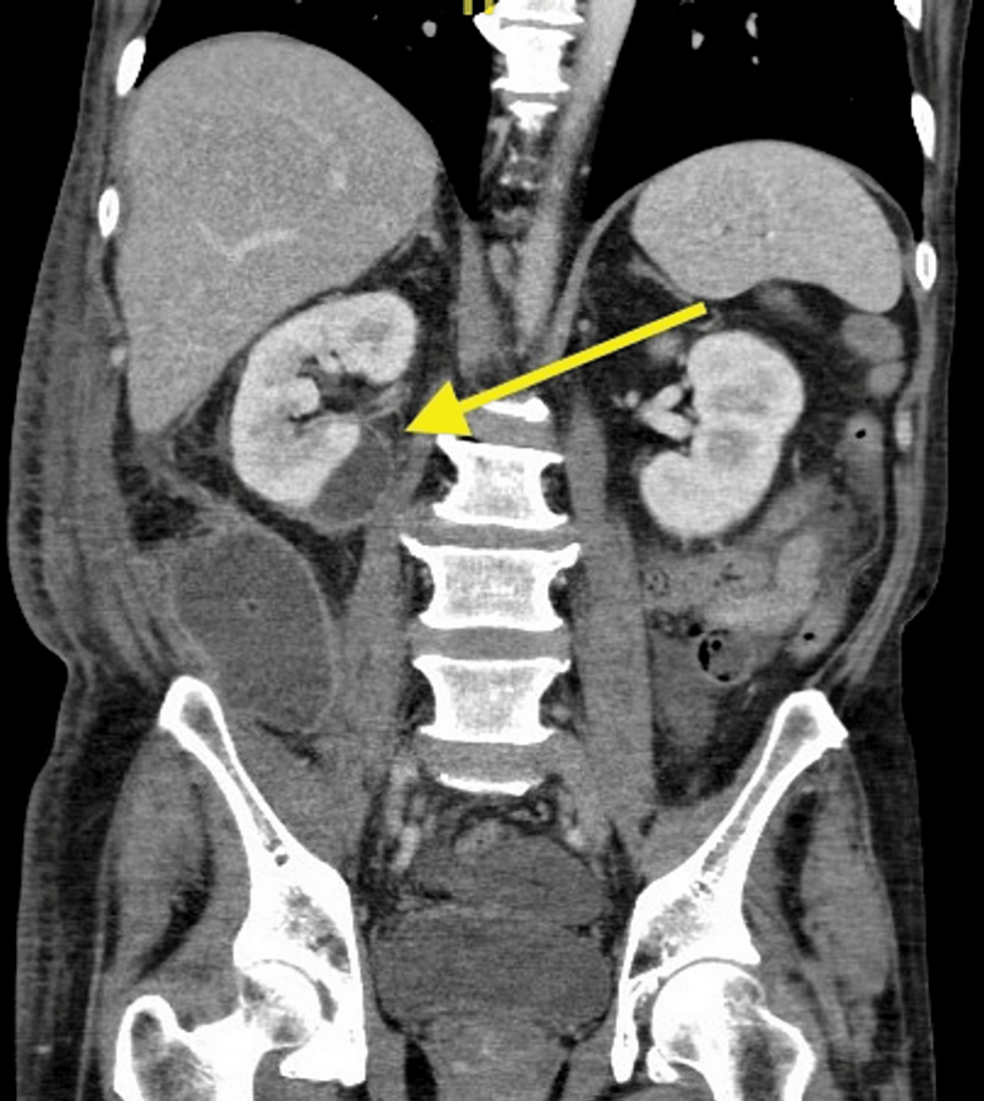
CT of the abdomen and pelvis The yellow arrow depicts a 2 x 6.4 x 4.3 cm abscess and capsular fluid surrounding the right medial inferior pole of the right kidney
